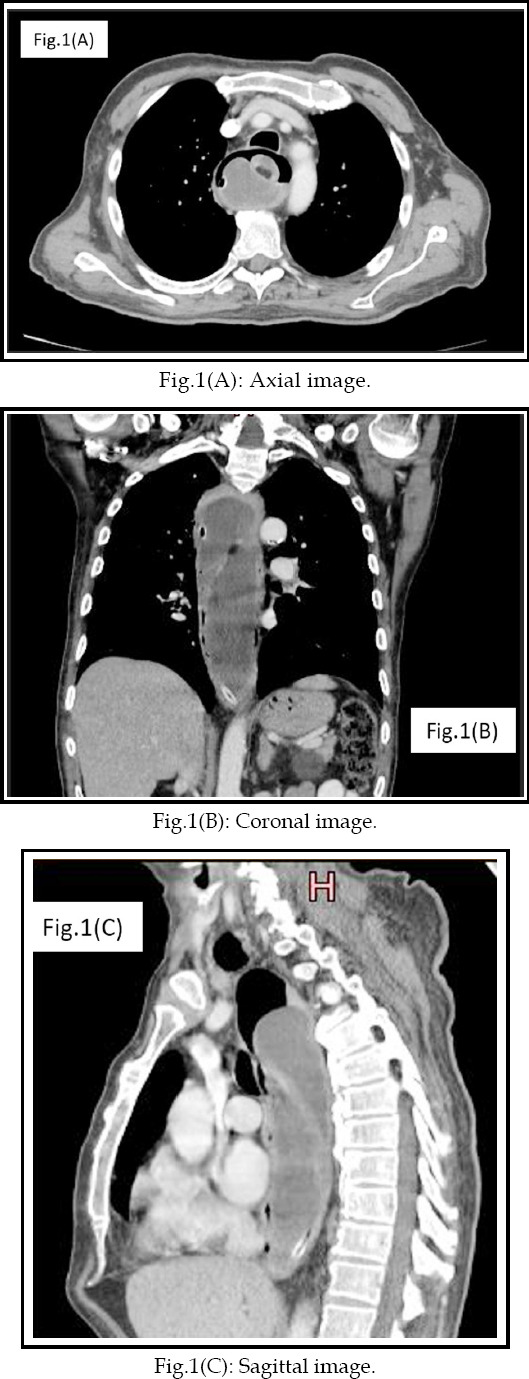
Findings of the contrast-enhanced computed tomography. Huge lobulated mass lesion in esophagus almost completely occupying its lumen. There is evidence of patchy intralesional fatty components. NG tube is also partially visualized.
